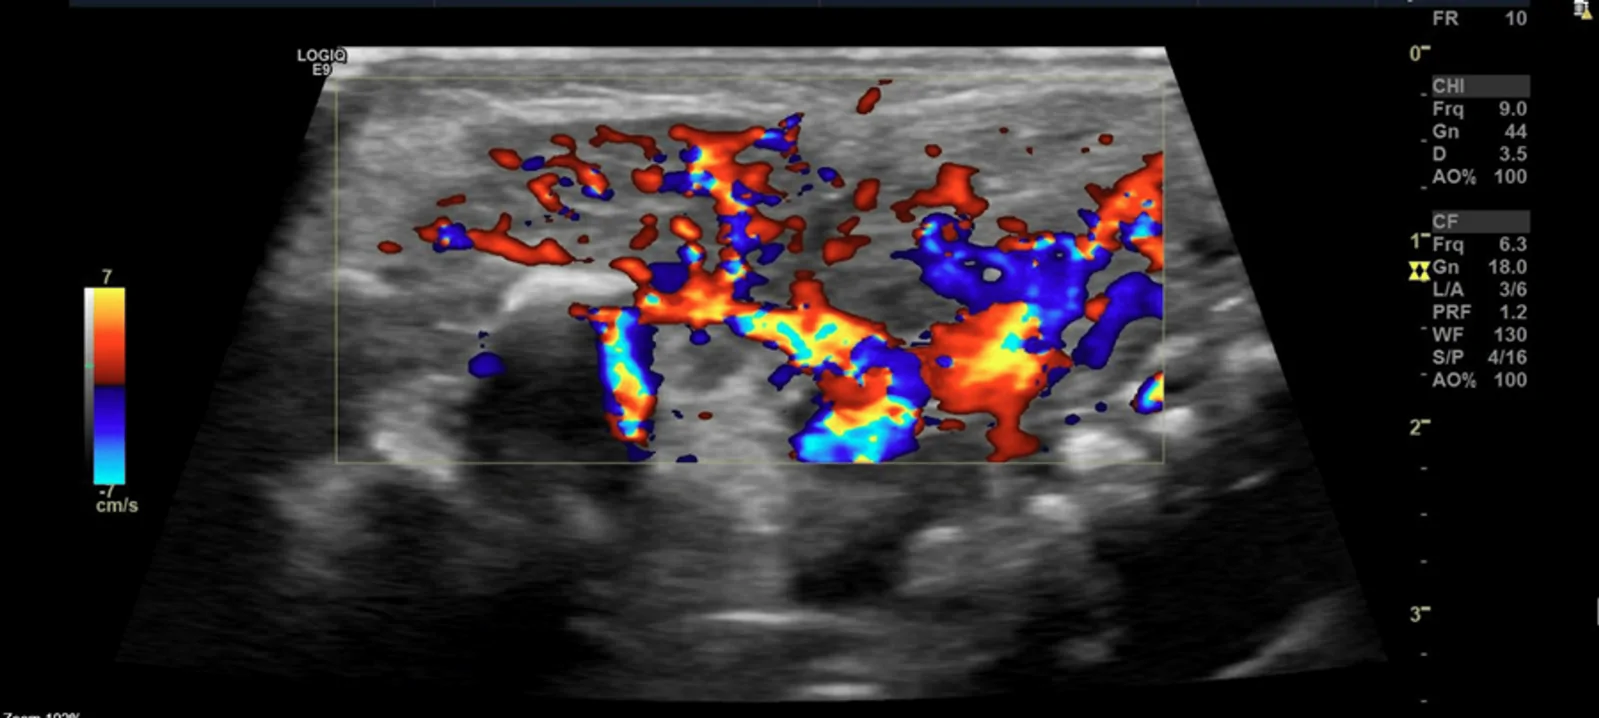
Ultrasound of the left parotid gland showing heterogeneous echotexture and increased vascularity on Doppler imaging, suggestive of early parotitis
